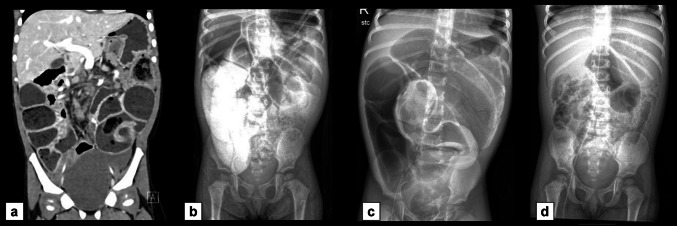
CT and small bowel follow-through images obtained in a 2-year-old girl with MMIHS who presented with abdominal pain and distension. (a) Coronal CT image with IV contrast shows diffusely dilated bowel loops without a transition point. (b) On the radiograph obtained 7 h after contrast administration, contrast remained within dilated bowel loops. (c) On day 9 after contrast administration, contrast continued to remain within dilated bowel loops. (d) Two weeks after contrast administration, contrast finally completely passed, and bowel loops were no longer dilated. The patient was managed for functional obstruction and bacterial overgrowth with bowel decompression using gastric suction
